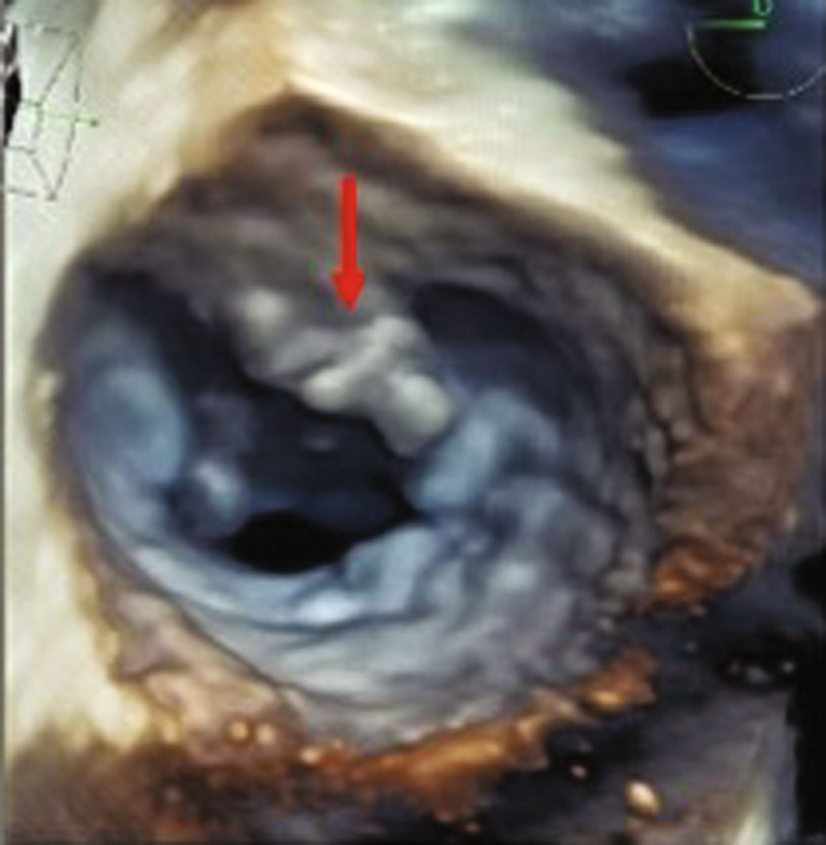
3D transesophageal echocardiography of infected TAVR showing bulky vegetation on the anterior mitral valve leaflet (arrow).
